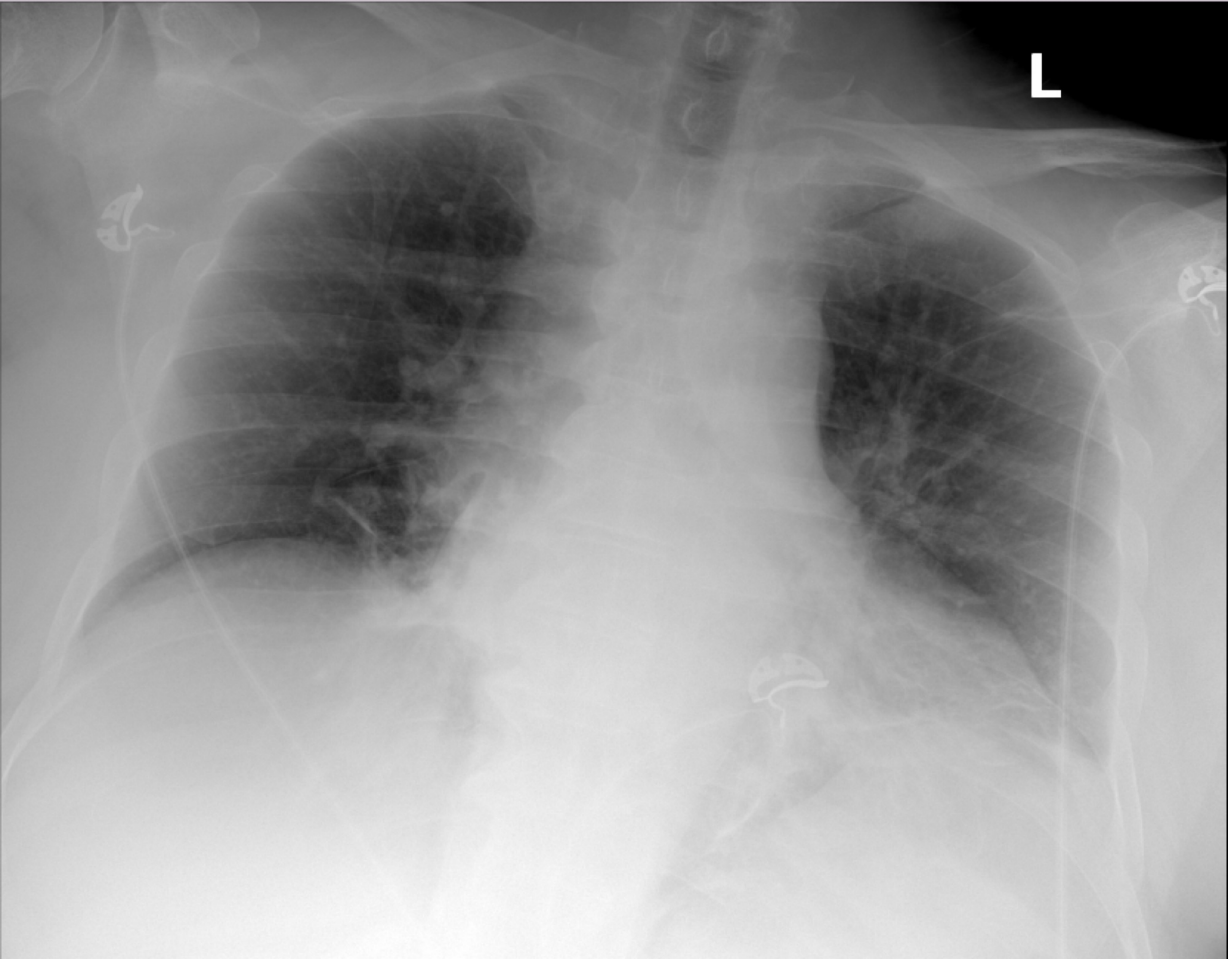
CXR showing left lung atelactasis/pleural effusion. CXR, chest X-ray
